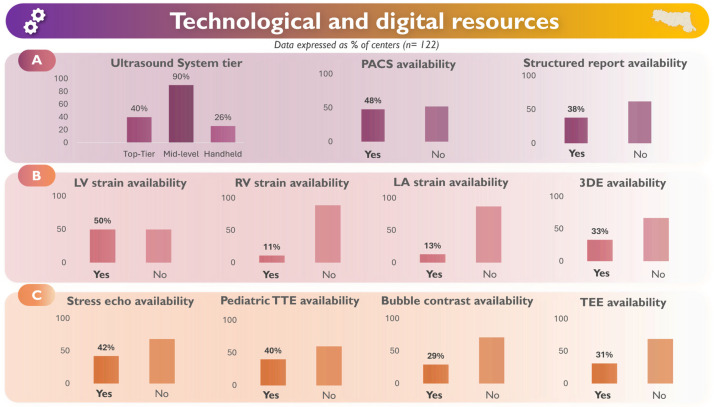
Technological and digital resources of echocardiography laboratories in Emilia-Romagna. Panel A: ultrasound systems and digital infrastructure. Panel B: advanced imaging tools. Panel C: advanced procedures. Data are presented as percentages of centers.
